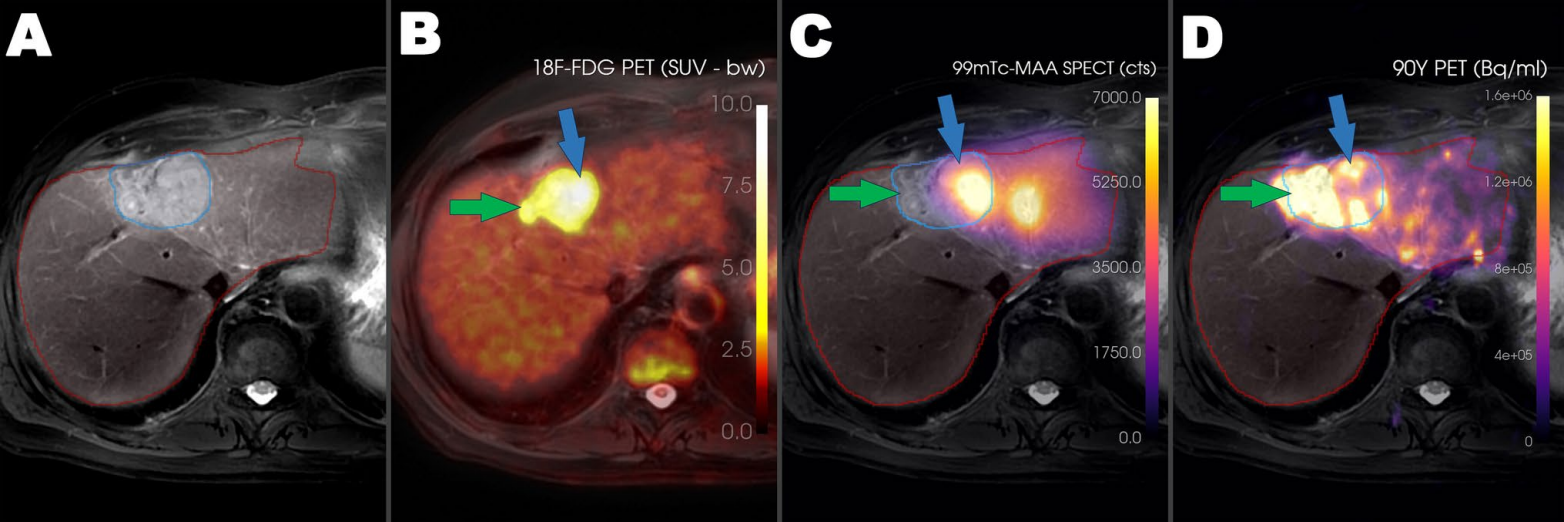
A 66-year-old man was treated for solitary metastasis of colorectal carcinoma. The lesion located in segment II-IVA of the liver was visualized on axial T2-weighted MR (A) and axial PET/MR fusion (B) images. Pre-treatment Tc-99m-MAA SPECT-MRI fusion images revealed intense uptake of Tc-99m-MAA in the segment II component of the lesion (C, blue arrow), while the non-perfused part of the tumor was also identified (C, green arrow). An additional dose was administered during treatment to ensure complete coverage of the tumor and could be visualized in post-treatment Y-90 PET/MR images (D). Consequently, the patient was excluded from the study.
